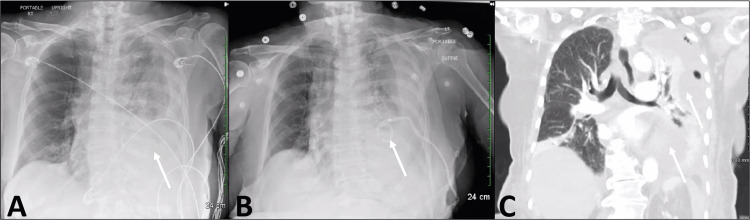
A). Anterior-posterior chest x-ray on admission showing a left-sided chest pleural effusion indicated by the white arrow. B). Hospital day 13 anterior-posterior chest x-ray showing minimal improvement after administration of tissue plasminogen activator and dornase alfa, white arrow indicating the pigtail catheter through which the tissue plasminogen activator and dornase alfa was given. C). Coronal segment of a computed tomography of the chest showing complete atelectasis and a left-sided hemothorax as indicated by the two white arrows.
